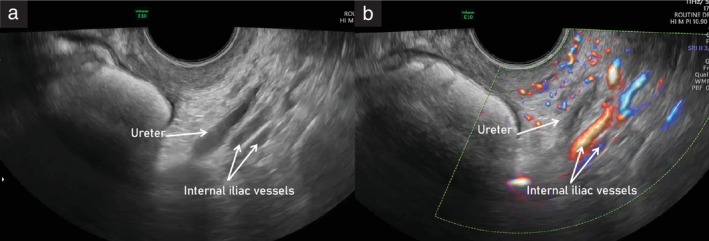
Ultrasound images showing course of the left ureter within the pelvic sidewall, running parallel to the internal iliac vessels, on grayscale (a) and power Doppler (b) imaging. The ureteric dilatation that can be observed in the grayscale image (a) is due to physiological peristalsis.
